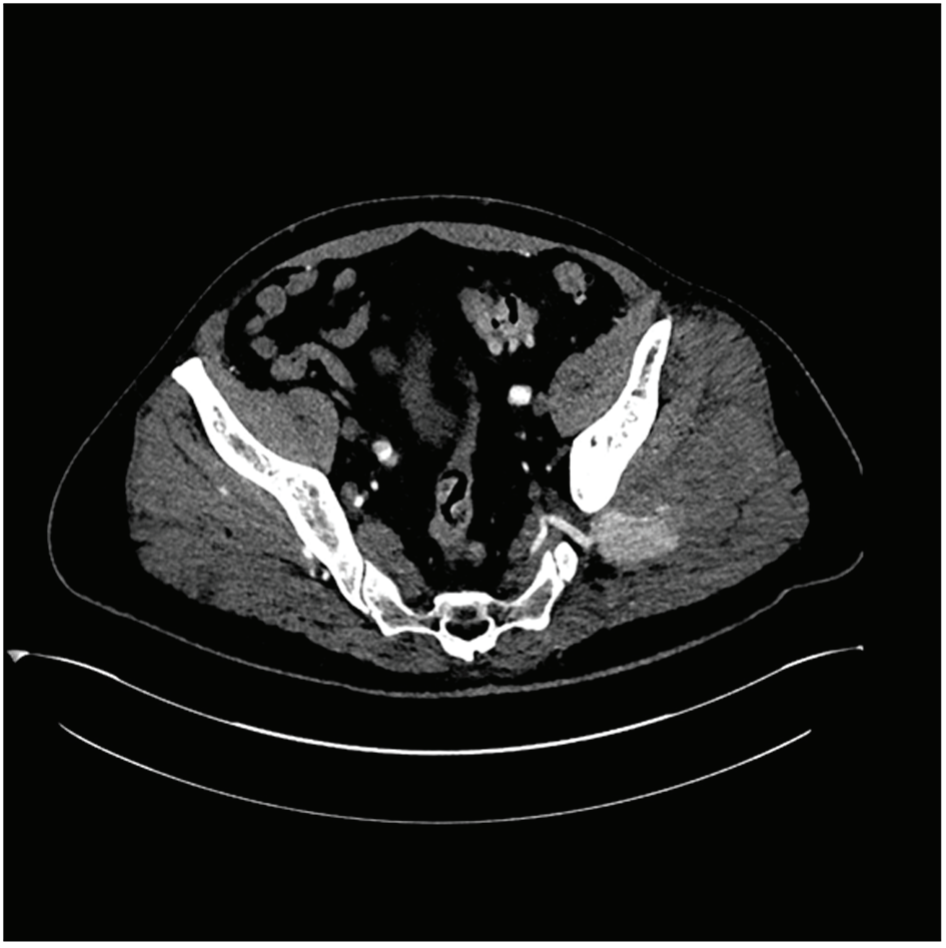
Contrast-enhanced computed tomography of the pelvis showing an area of contrast extravasations in the left gluteus medius muscle.
